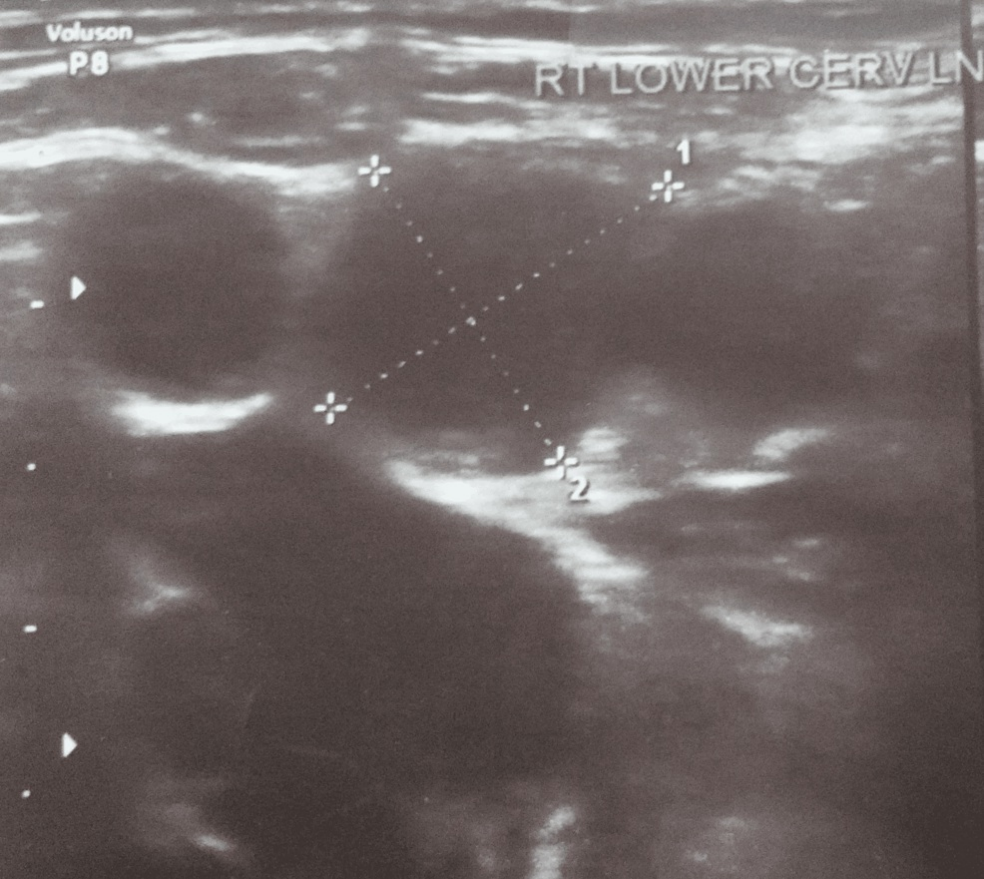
An ultrasound of the neck suggestive of enlarged right cervical lymph nodes.
